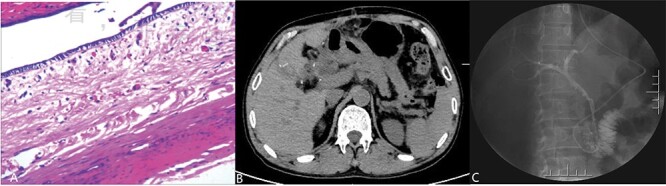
(Postoperative image) A: Pathological examination revealed a cyst with a fibrous wall, dense chronic inflammatory infiltration; B: CT after operation; C: Radiography through nose-bile tube after operation.
